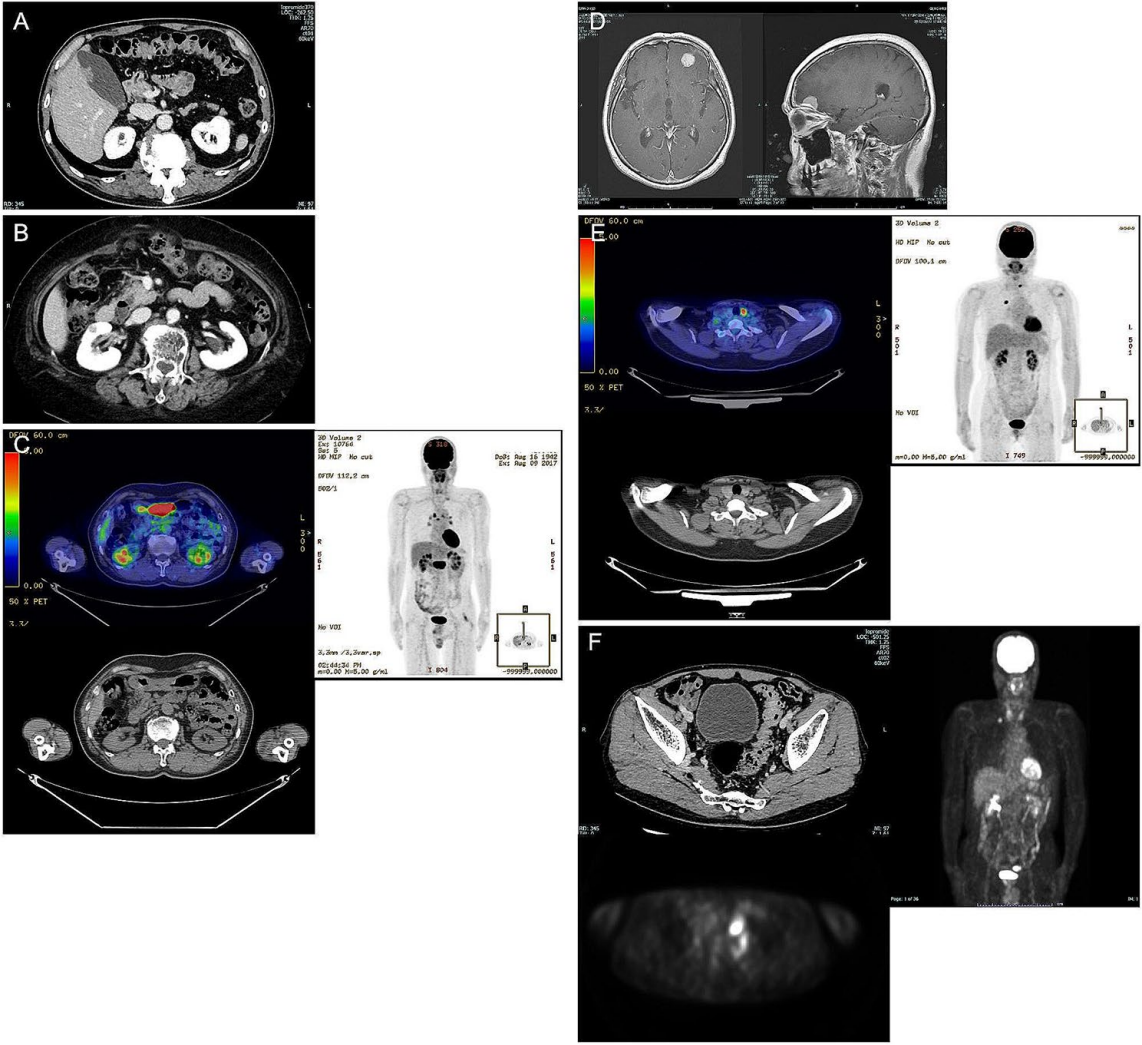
Incidental findings of treatable malignant potential tumor during preoperative imaging (A) Case 4 (71-year-old male with bilateral GGNs in right S1 and left S1 + 2): Gallbladder cancer was detected on contrast-enhanced (CE)-CT. CT revealed 18 mm pedunculated tumor without invasion into the liver parenchyma. Case 4 received extended cholecystectomy before pulmonary resection. (B) Case 7 (86-year-old female with right S6 GGN): Renal cancer was detected on CE-CT and PET/CT. The 10 mm nodule showed hyperenhancement in the arterial phase and washout in the equilibrium phase of CE-CT. Case 7 was followed up with CT after pulmonary resection. (C) Case 8 (75-year-old male with right S7 GGN): Gastric cancer was detected on PET/CT. PET/CT showed anterior gastric wall thickening and high maximum standardized uptake value (SUV_max_ 27.9) in the pyloric end of stomach. After pulmonary resection, Case 8 received distal gastrectomy with D2 lymph node dissection and the final pathological stage was IIIB. (D) Case 9 (77-year-old female with left S1 + 2 GGN and left breast cancer 5.5 cm, pT1cN0): Meningioma was detected on brain MRI. The enhancing brain mass adjacent to the anterior cranial base was 20 mm, with edematous. Case 9 was followed up with CT after pulmonary resection. Case 9 had no tumor-related symptoms. (E) Case 17 (56-year-old female with right S1 solid adenocarcinoma): Thyroid papillary carcinoma was detected on PET/CT. The low-density nodule showed SUV_max_ 7.0. Case 17 received thyroidectomy after pulmonary resection (F) Case 18 (68-year-old male with right S1 solid adenocarcinoma): Sigmoid colon cancer was detected on CE-CT and PET/CT (conducted at another hospital). PET/CT revealed sigmoid colonic wall thickening and high FDG uptake. Case 18 received laparoscopic sigmoidectomy with D2 lymph node dissection before pulmonary surgery
